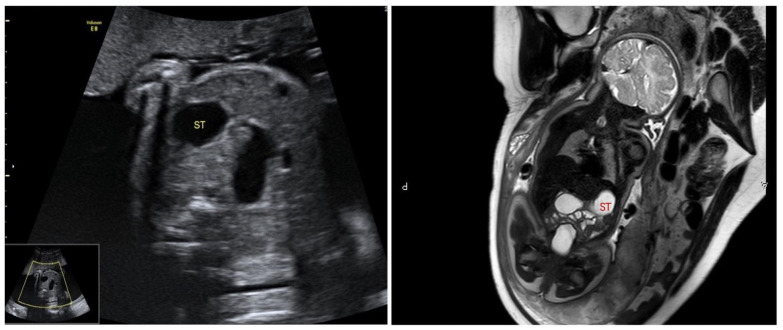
“Double-bubble” sign in ultrasound and MRI examination.
